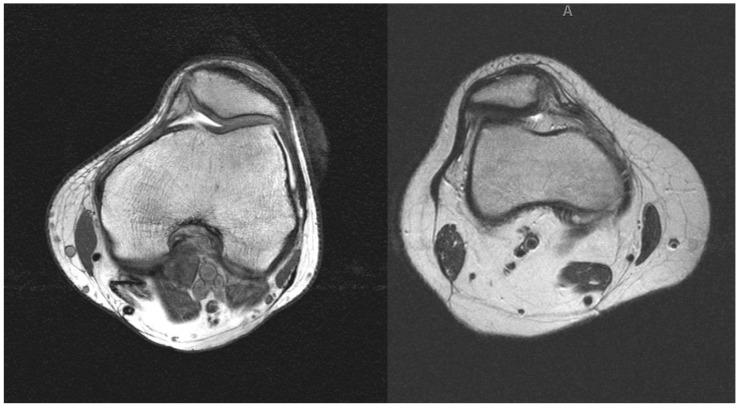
Evidence of lateral patellar compression syndrome (LPCS) at MRI.
